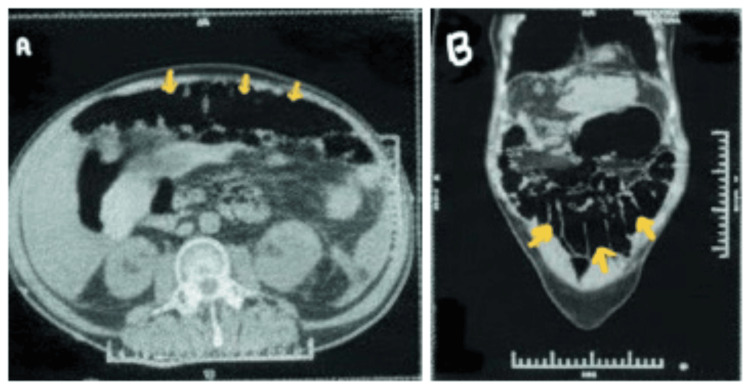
NCCT Abdomen axial and coronal images (A and B) showing dilated large-bowel loops (yellow arrows) without evidence of a transition point, suggestive of acute colonic pseudo-obstruction. NCCT: Non-contrast computed tomography
